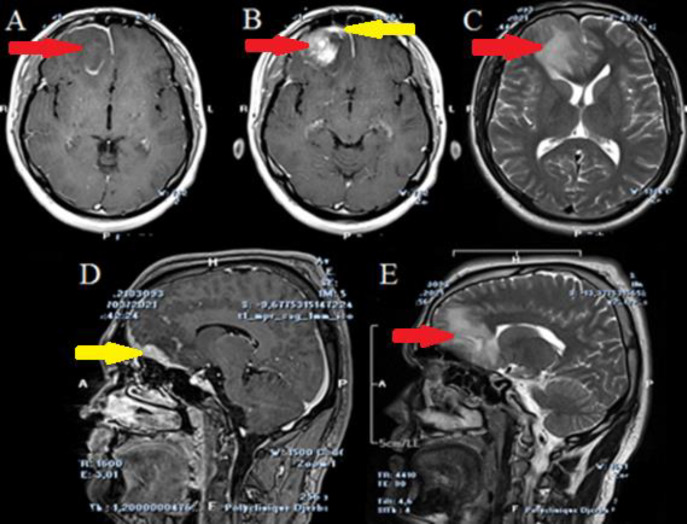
Axial section (A, B, C) and sagittal section (D, E) of a brain MRI on T1-WI without Gadolinium (A), with Gadolinium (B,D), T2-WI (C, E) of a preoperative MRI showing a right frontal lesion with a heterogeneous enhancement surrounded by finger like edema. Sagittal reconstructions show right fronto-basal pachymeningitis
